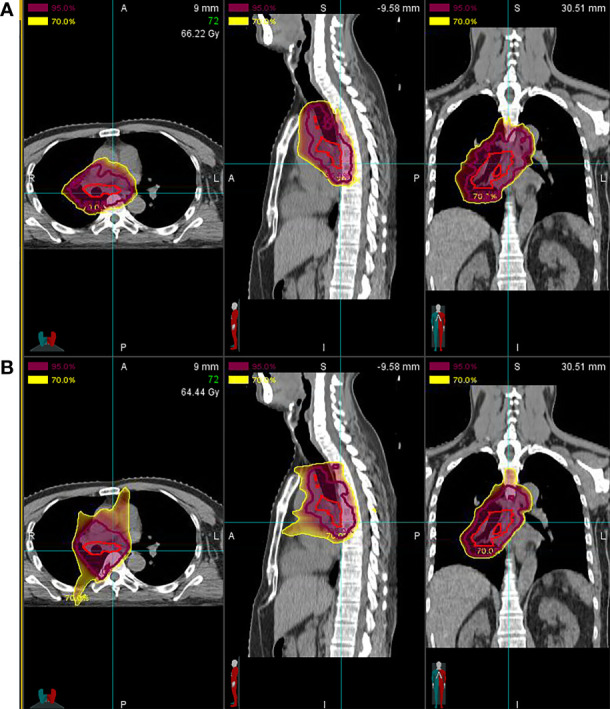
Comparison of treatment planning for particle *vs*. X-ray radiotherapy (RT). (A) Proton radiotherapy (PRT) and carbon-ion radiotherapy (CIRT) show a significant decrease in the average doses to the heart and lungs compared with (B) X-ray RT [mean heart dose, 13.95 gray equivalent to photon (GyE) *vs*. 16.40 Gy; mean lung dose, 9.12 GyE *vs*. 21.46 Gy].
